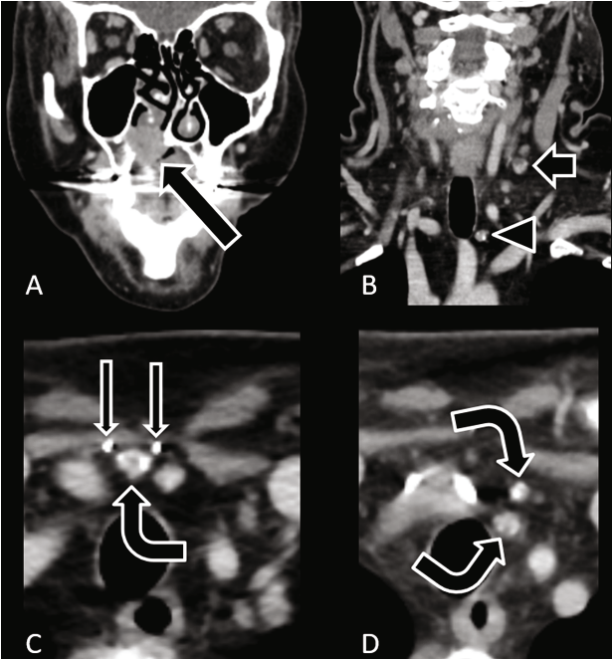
A 60‐year‐old female with a history of papillary thyroid carcinoma (PTC) in remission after treatment 20 years prior presented with biopsy‐proven adenoid cystic carcinoma (ACC) of the palate. (A) Coronal contrast‐enhanced soft tissue neck CT shows an aggressive enhancing soft tissue mass centered on the right hard palate with erosion into the nasal cavity (arrow). (B) Coronal image of the neck shows a necrotic left Level III node (short arrow), which would be atypical for metastatic disease from a right palate mass, and a Level VI node with internal calcifications (arrowhead). (C, D) Axial images of the lower neck reveal an absent thyroid with surgical clips (thin arrows) and multiple calcified lymph nodes (curved arrows). Nodal calcifications are most common in metastatic thyroid carcinomas. Palate mass resection showed ACC, and neck dissection showed metastatic PTC in multiple nodes, including in Level VI and left Level III.
